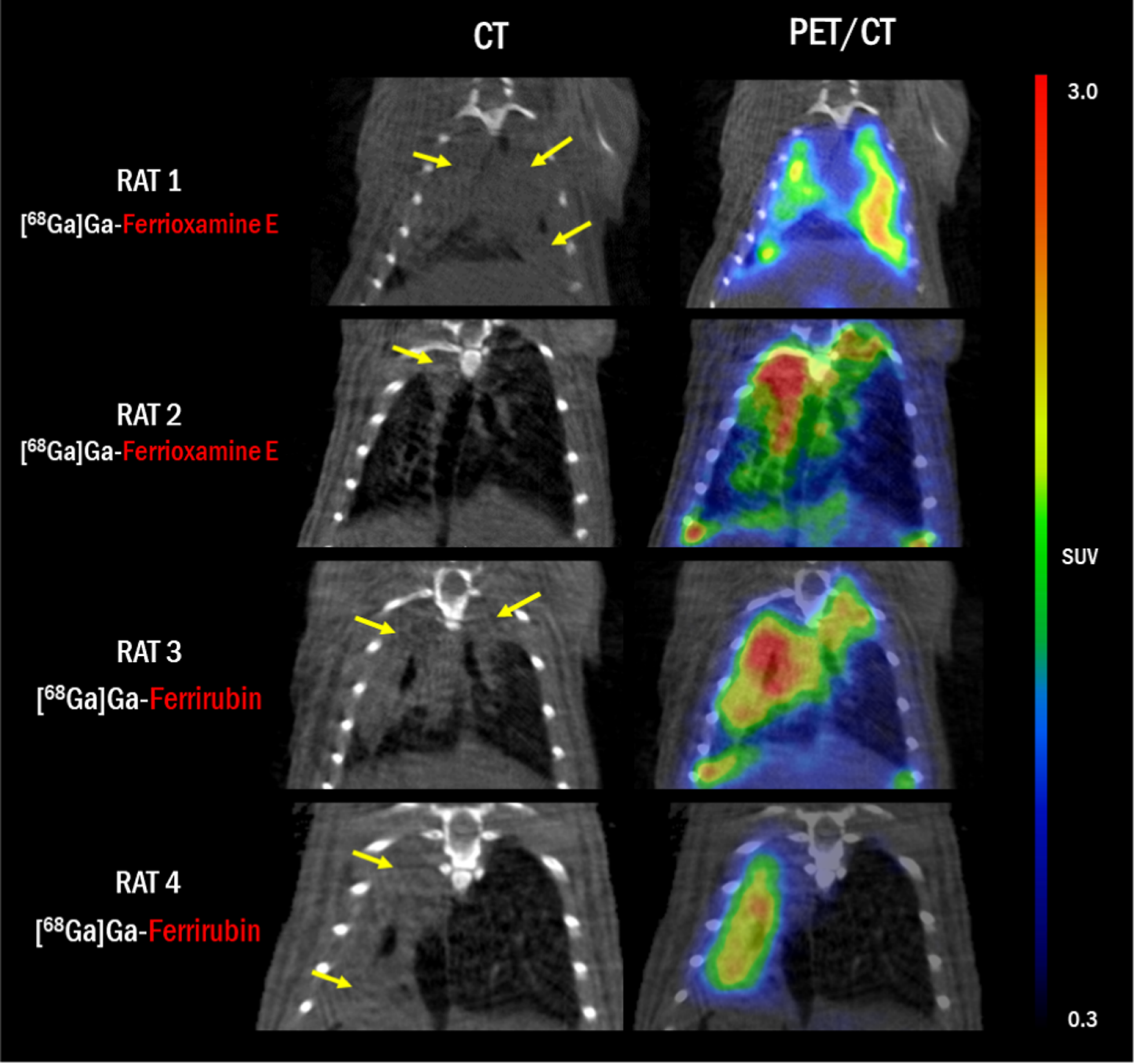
Detailed CT and PET/CT images of coronal sections of lungs
